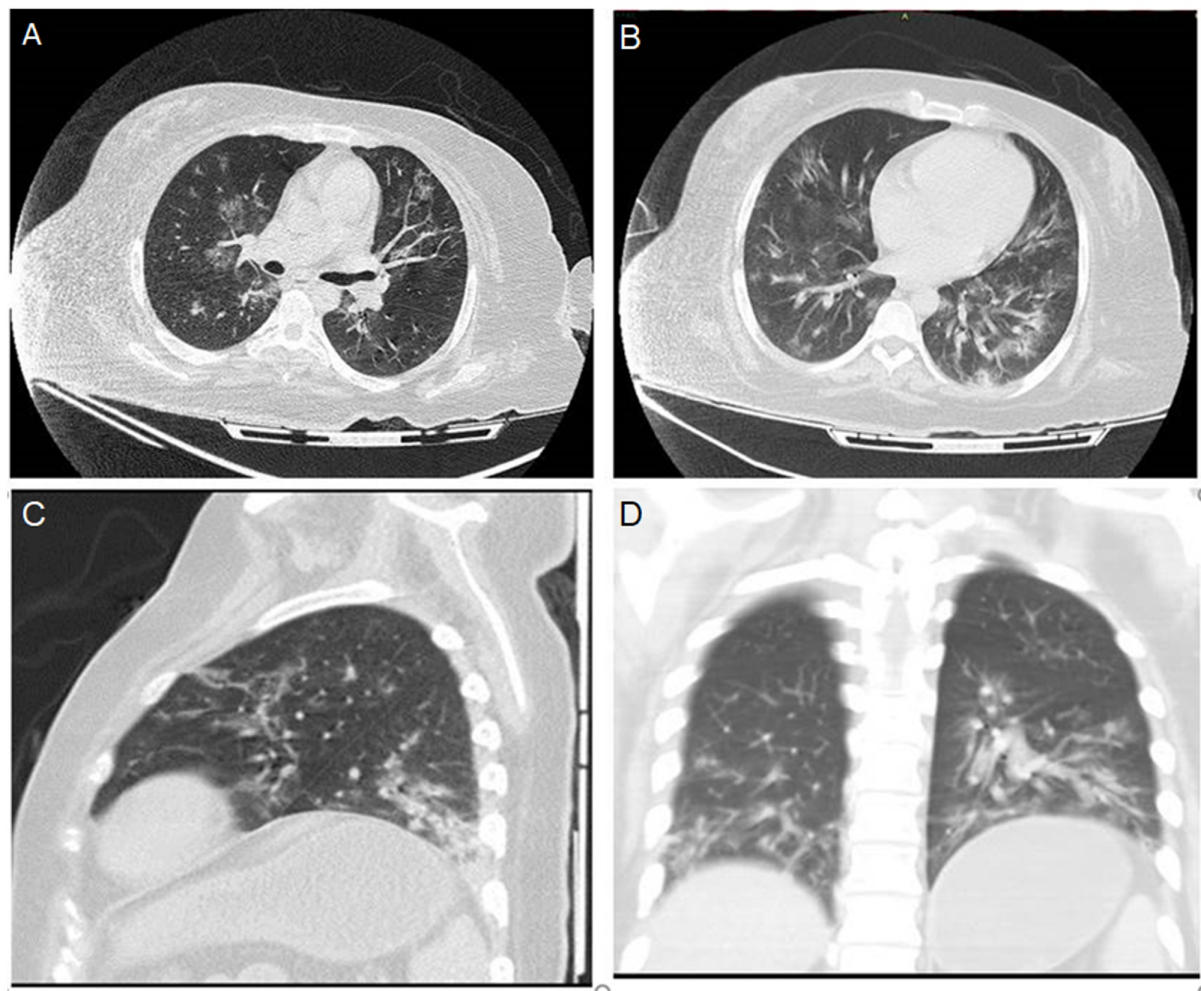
CT of the chest with multiple areas of increased density in a ground-glass pattern with diffuse bilateral distribution with peripheral predominance coexisting with consolidation in lower lobes and intralobular septal thickening. Axial mainstem bronchii level (A), Axial right pulmonary artery level (B), Sagittal (C), Coronal (D)
